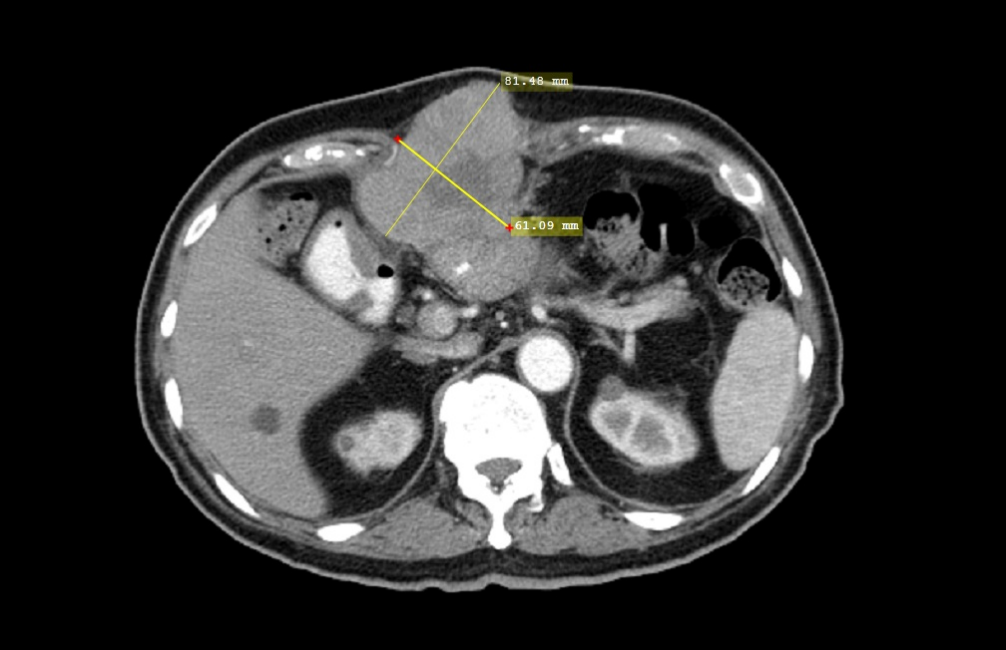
CT Abdomen Before Treatment With Pembrolizumab Axial contrast enhanced CT of the abdomen showing an 8 x 6 cm mass with central necrosis consistent with recurrent HCC.
